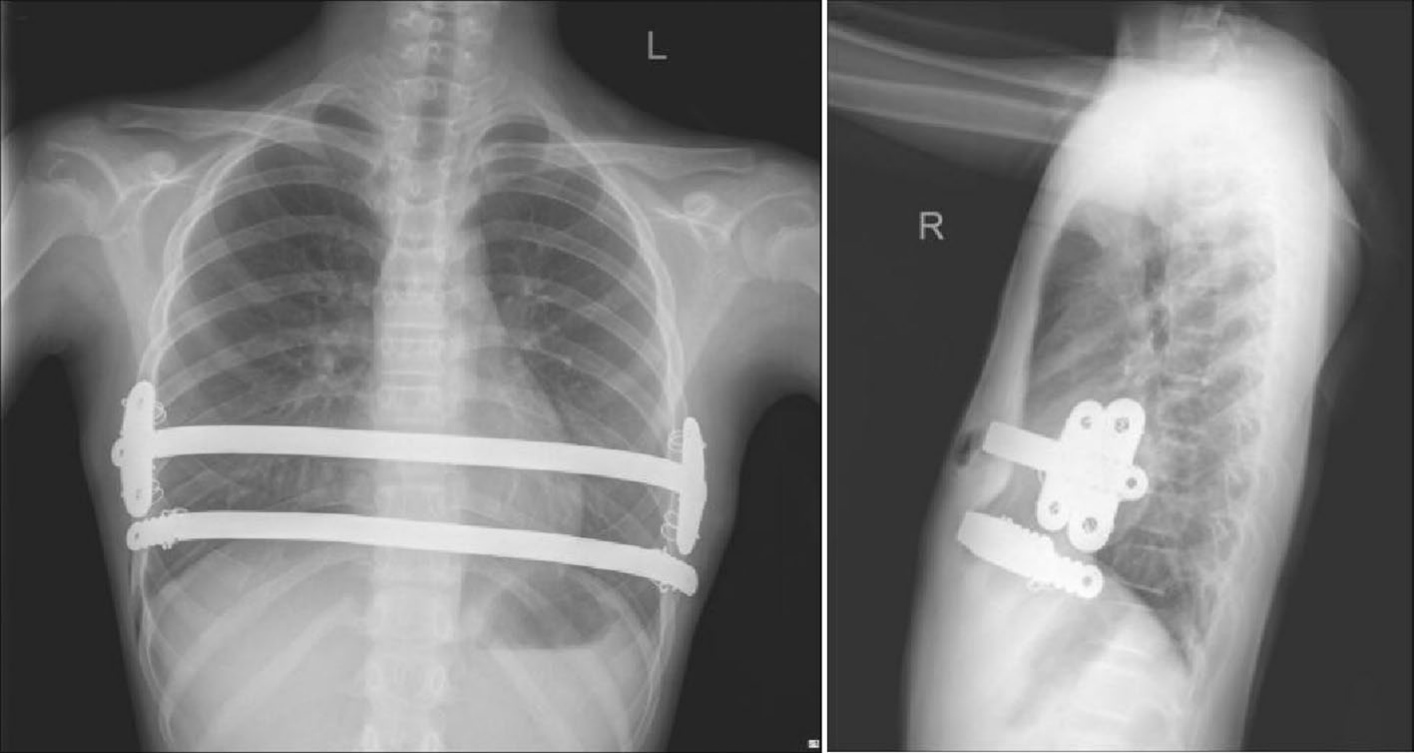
Postoperative frontal and lateral radiographic films of the chest, showing that the sternum is flat and straight, and the plate and fixing pieces are well-positioned.
